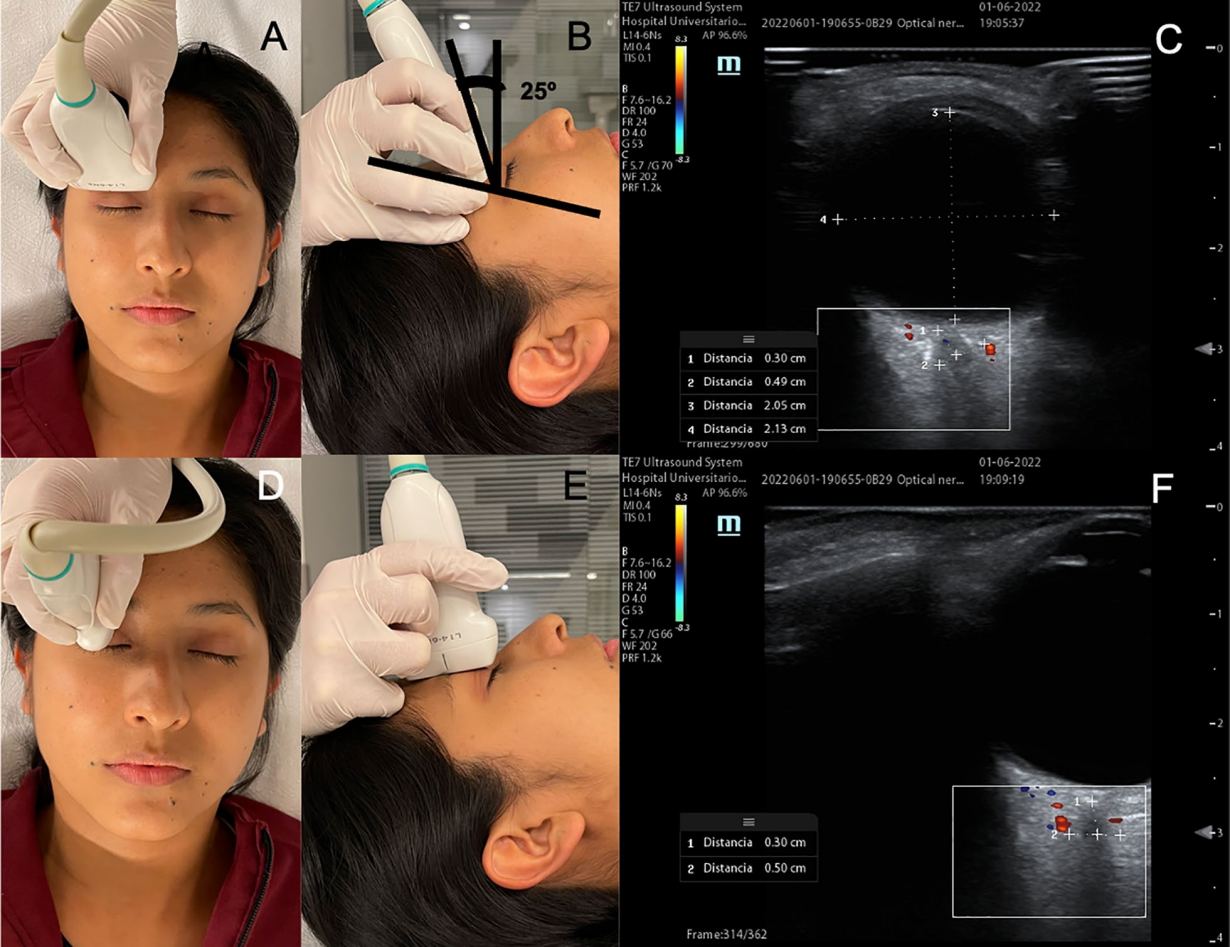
Ultrasound measurements technique. A–B: The transducer positioned on the transverse axis with an initial angle of 25° and tilted until the optic nerve and the papilla were visualized. C: Optic nerve and papilla with color doppler mode showing the central vessels of the retina, the ciliary vessels and measurement performed using central vessels of the retina direction and landmarks from the ciliary vessels. D–E: The transducer positioned on the vertical axis. F: Optic nerve and papilla with color Doppler mode showing the central vessels of the retina, the ciliary vessels and measurement performed using central vessels of the retina direction and landmarks from the ciliary vessels
